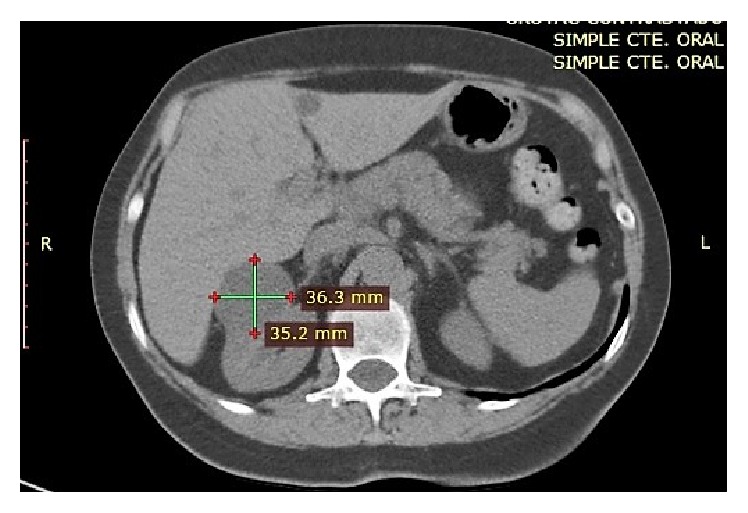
Noncontrast CT scan: water density renal mass, compatible with a simple renal cyst.
